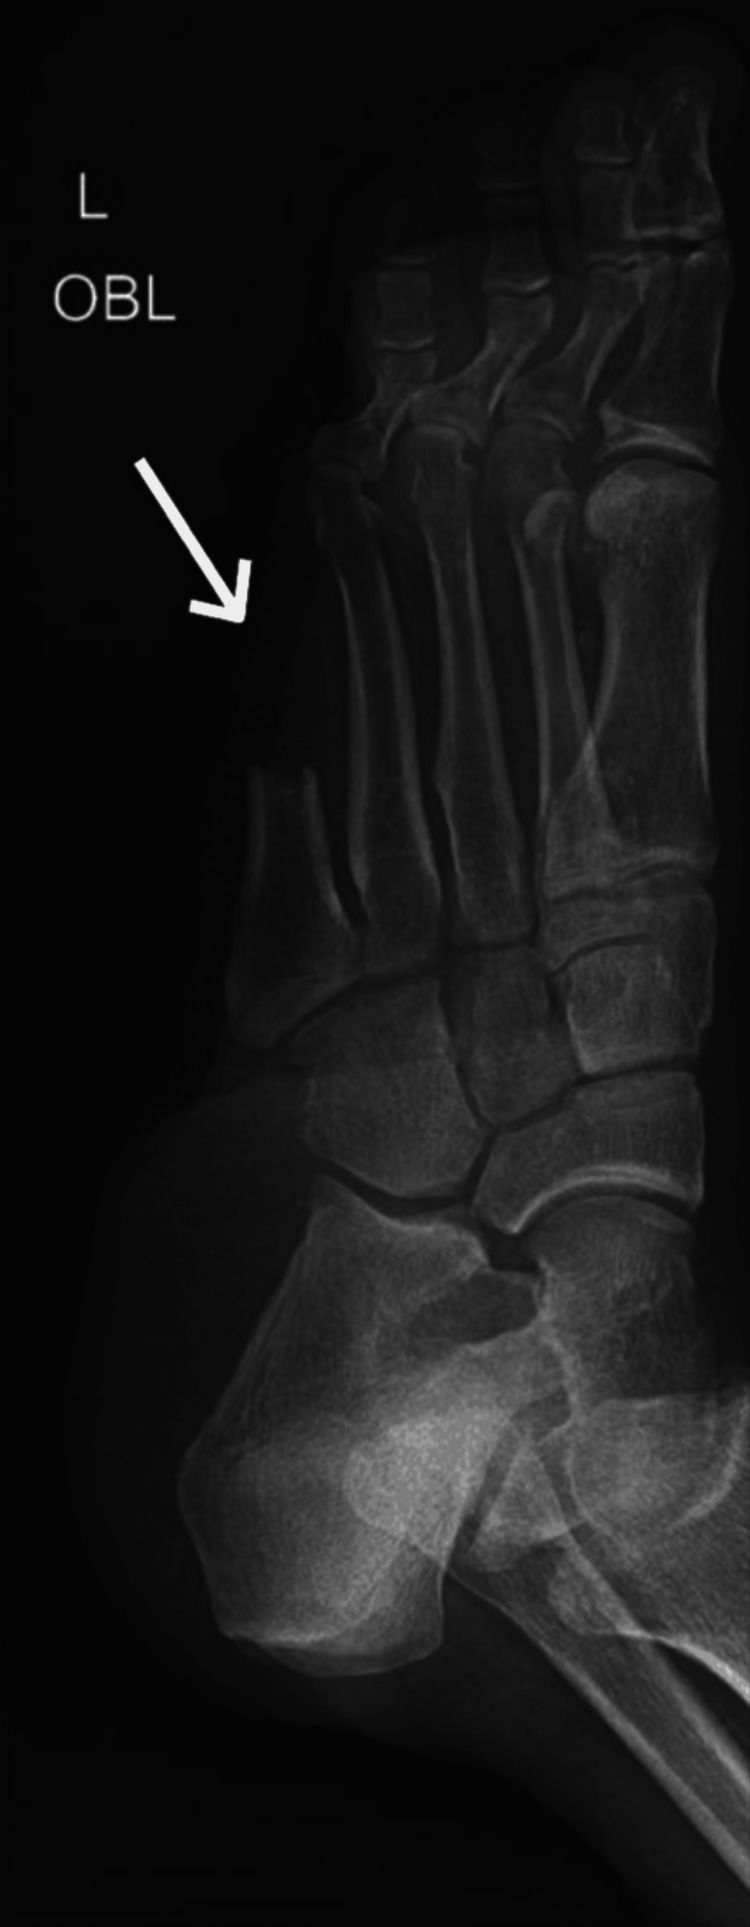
Oblique radiograph of the left foot demonstrating prior surgical changes with non-visualization of the fifth toe phalanges and truncation of the distal fifth metatarsal
